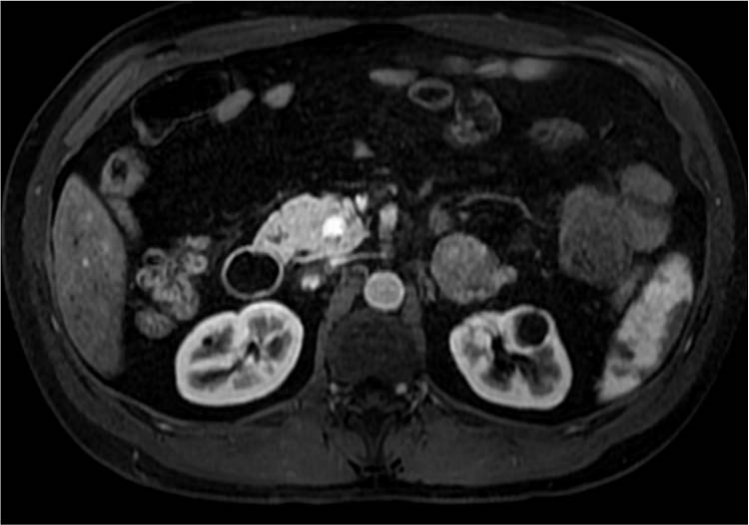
CT scan in the arterial phase after contrast injection. The highly hyperintense 13 mm cephalopancreatic hypervascular nodule characteristic of neuroendocrine tumors is clearly noticeable. In the lateral lip of the left adrenal gland, a 39 mm nodule with clear margins showing strong T1 signal reduction out of phase and inhomogeneous, weak, enhancement after contrast medium (typical of adrenal adenomas) can be seen.
